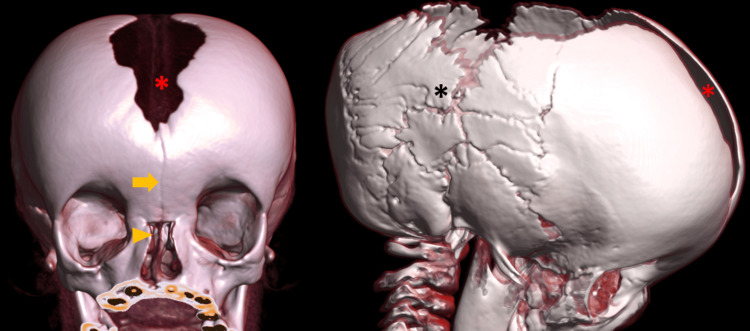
Volume-rendered CT images of the skull showing multiple wormian bones (black asterisk), persistent metopic suture (arrow), frontal bossing, absent nasal bones (arrowhead), and a wide-open sagittal suture and anterior fontanelle (red asterisk).
